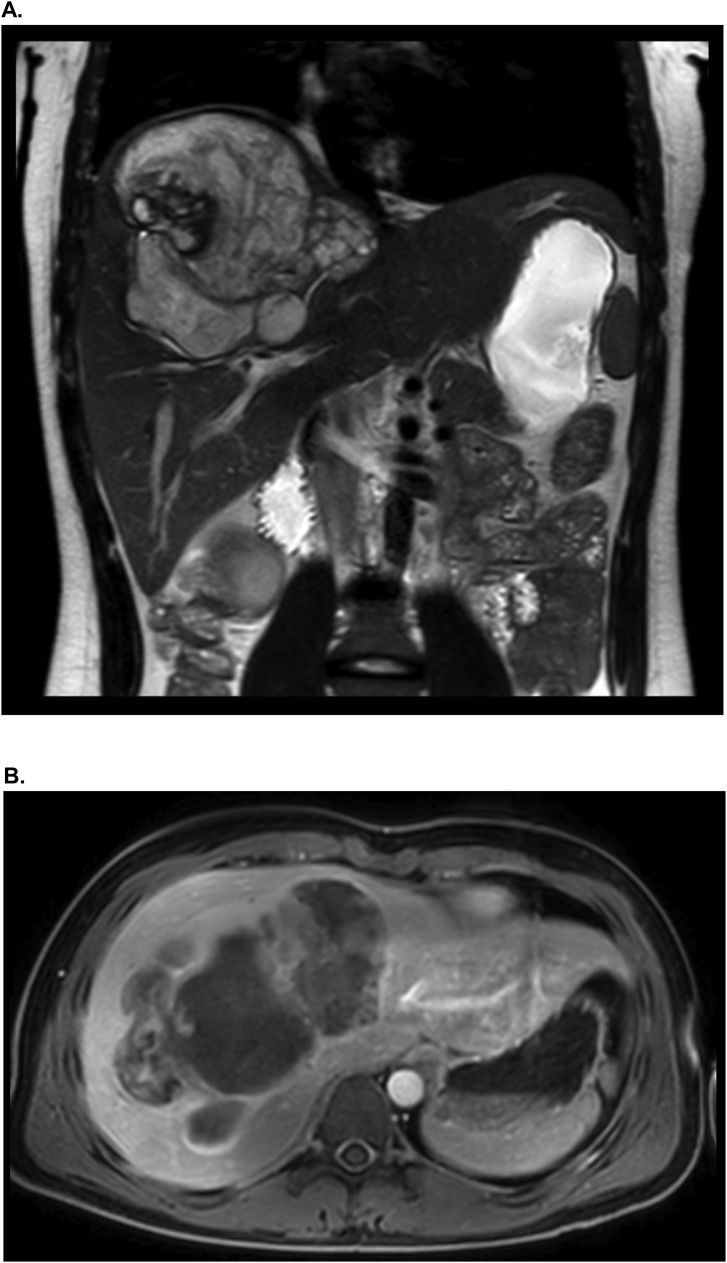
Preoperative MRI shows a heterogenic tumor mass in the right liver lobe before chemotherapy. The lesion invades segment IVa and V-VIII. A: Coronal MRI B: Axial MRI.
